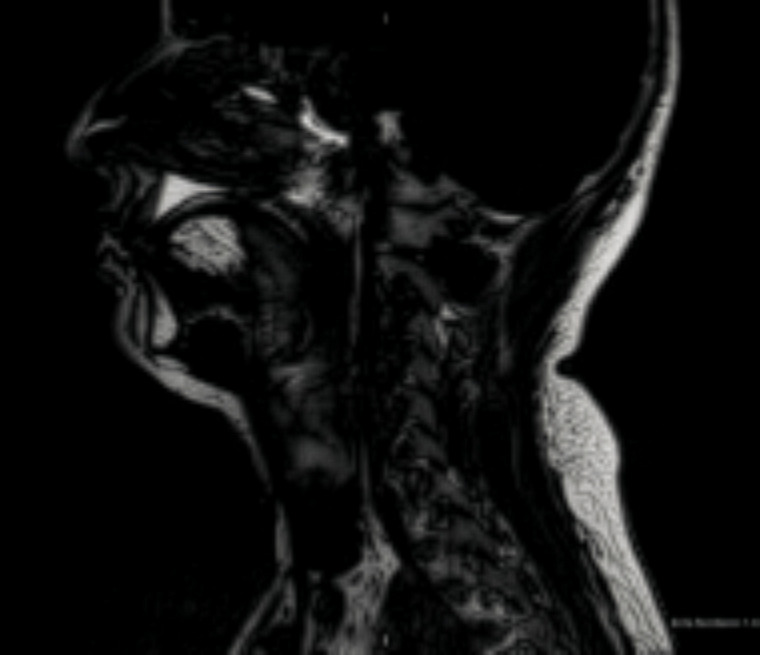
MRI Neck sagittal (arrow pointing to tongue base tumour).
